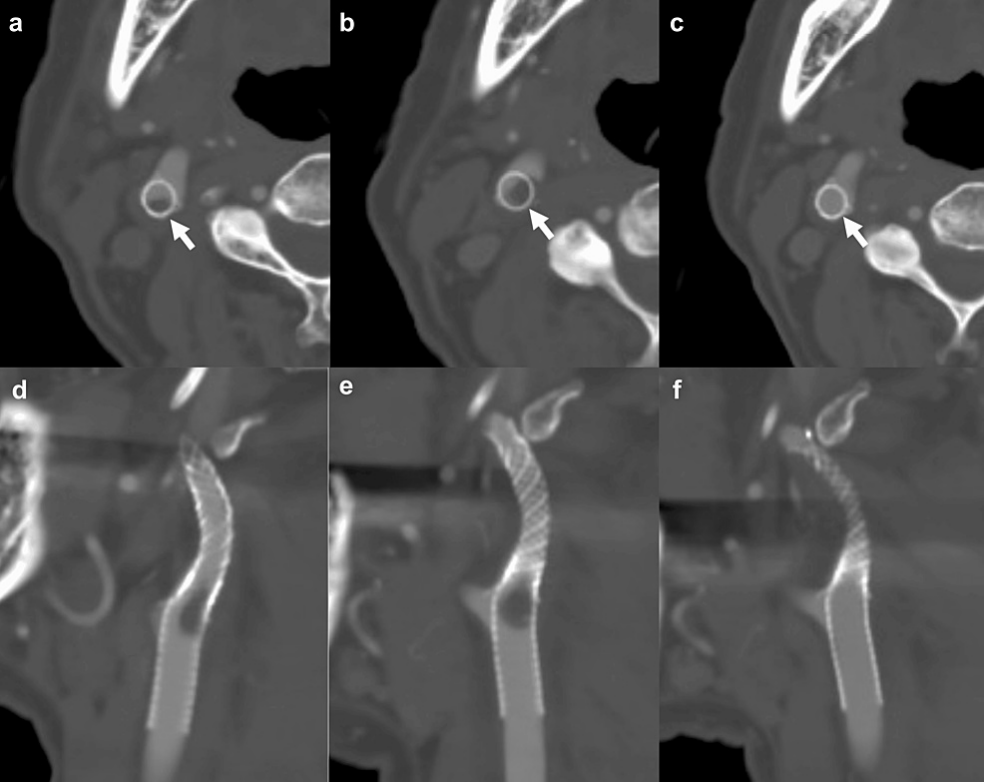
Chronological change in in-stent thrombosis in the right ICA (a), (d) CTA in the axial and sagittal plane four days after admission shows in-stent thrombosis at the origin of the right ICA (white arrow). (b), (e) CTA in the axial and sagittal 11 days after admission shows increasing in-stent thrombosis despite the addition of heparin (white arrow). (c), (f) CTA in the axial and sagittal plane 25 days after admission shows the disappearance of in-stent thrombosis due to increasing hydroxyurea dosage and the administration of aspirin (white arrow). ICA, internal carotid artery; CTA, computed tomography angiography
